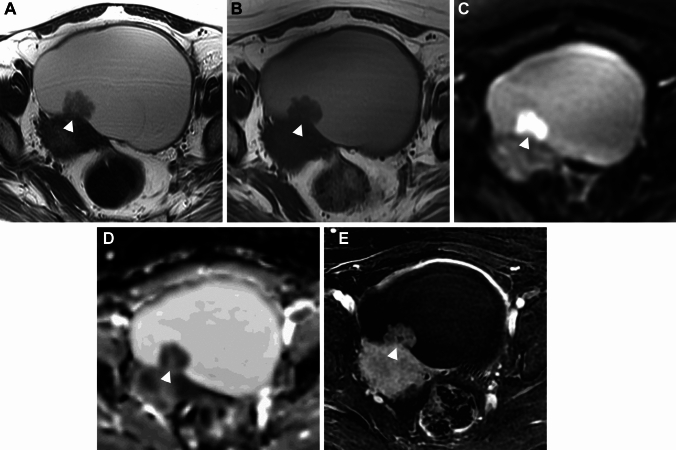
Endometriosis-associated ovarian carcinoma: clear cell carcinoma. A 52-year-old with suspicion of an ovarian cystic mass with mural nodules on ultrasonography examination. A T2-weighted image, B T1-weighted image, C DWI (*b* = 800 s/mm^2^), D ADC map, and E contrast-enhanced subtraction image show a left ovarian cystic mass with a mural nodule (arrowhead). The cyst contents show high signal intensity on both T1- and T2-weighted images reflecting hemorrhagic fluid. The mural nodule shows intermediate signal intensity on T2-weighted image and water diffusion restriction on DWI, and intense contrast-enhancement on post-contrast images clarified on the subtraction image
